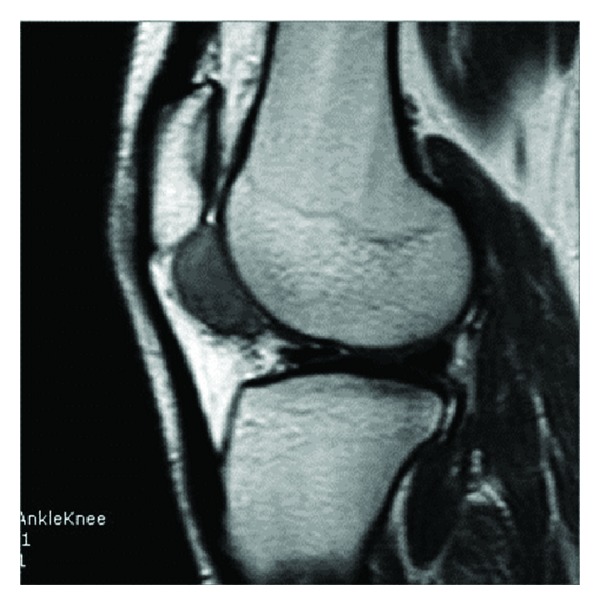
Hypointense lesion in infrapatellar region on T1-weighted MRI sequences.
